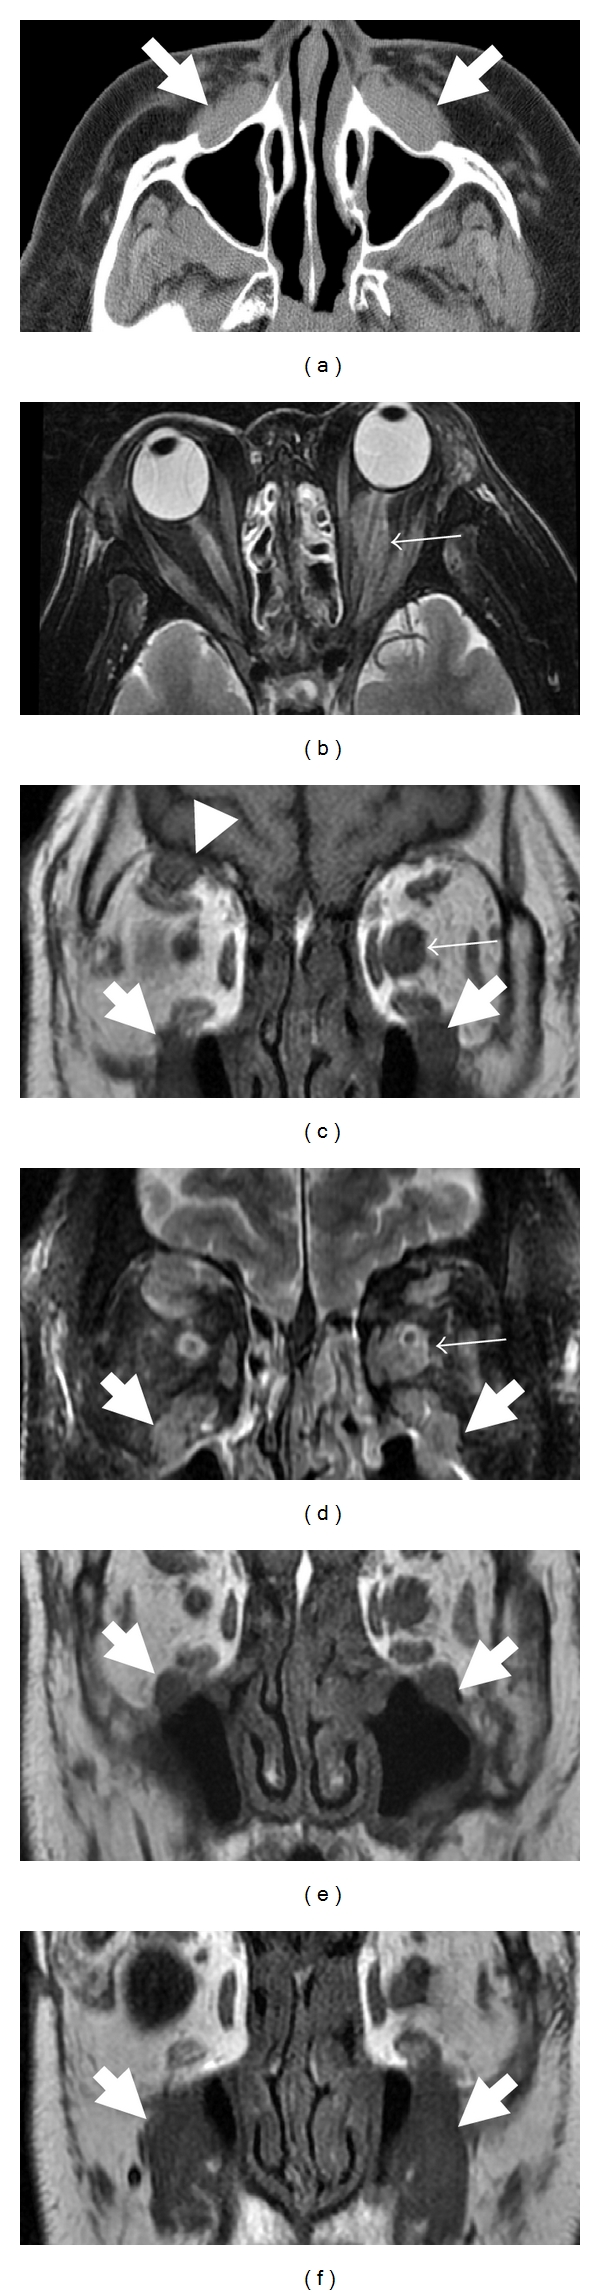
CT (a) and MRI ((b and d): T2-weighted images; (c, e, and f): T1-weighted images) of orbital space in 55-year-old man (case 1). CT image reveals soft tissue around the bilateral infra orbital nerve (a; arrows). MRI shows soft tissue around the left orbital nerve (b–d; small arrows), bilateral infraorbital nerve (c–f; arrows) and right supraorbital nerve (c and d; arrowheads). Left orbital nerve can be detected in the lesion (b and d).
